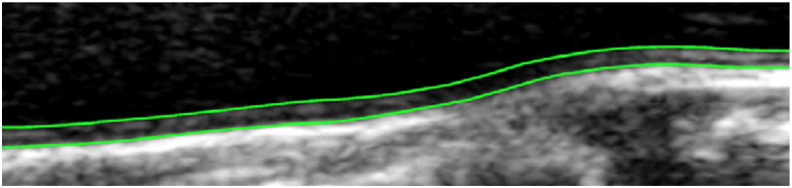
Detection of intima-media thickness in ultrasound carotid artery image.
